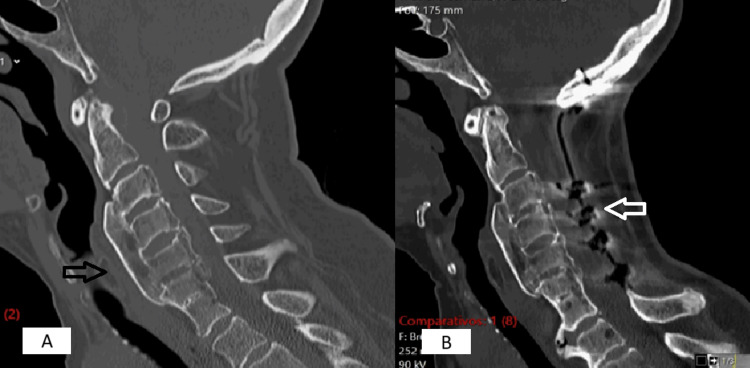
Comparison between pre- and post-operative computed tomography scans. (A) CT before surgery. The black arrow shows C3-C6 diffuse idiopathic skeletal hyperostosis. (B) CT after surgery. The white arrow shows a wide decompression of the cervical spinal cord
